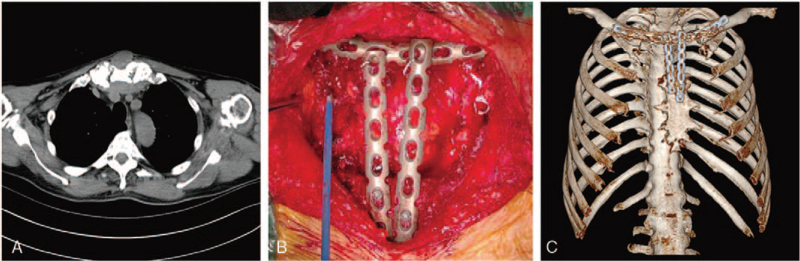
(A) Chest CT scanning displayed an anterior chest wall neoplasm. (B) The second operation of tumor resection and chest wall reconstruction. (C) CT review after the second operation.
